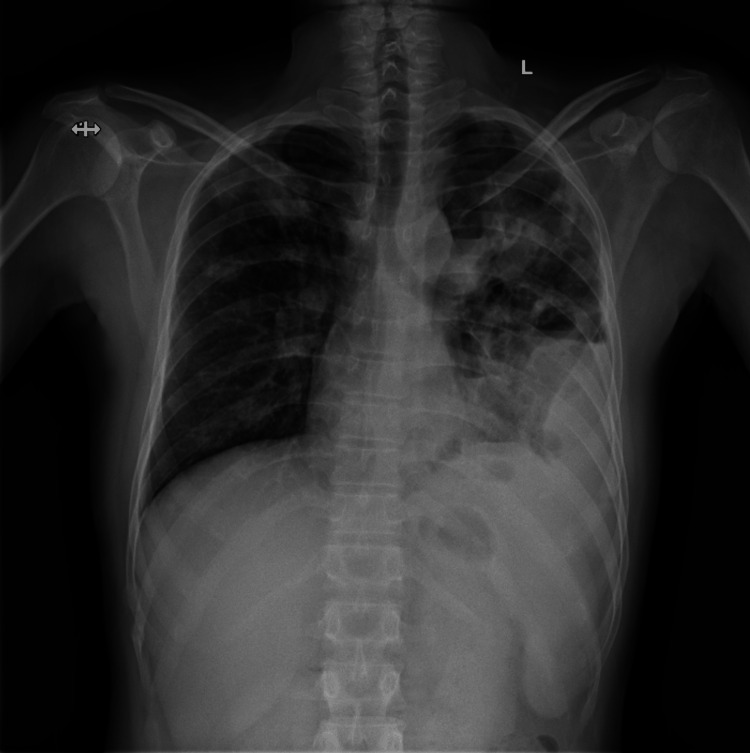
Chest X-ray shows bilateral consolidation
